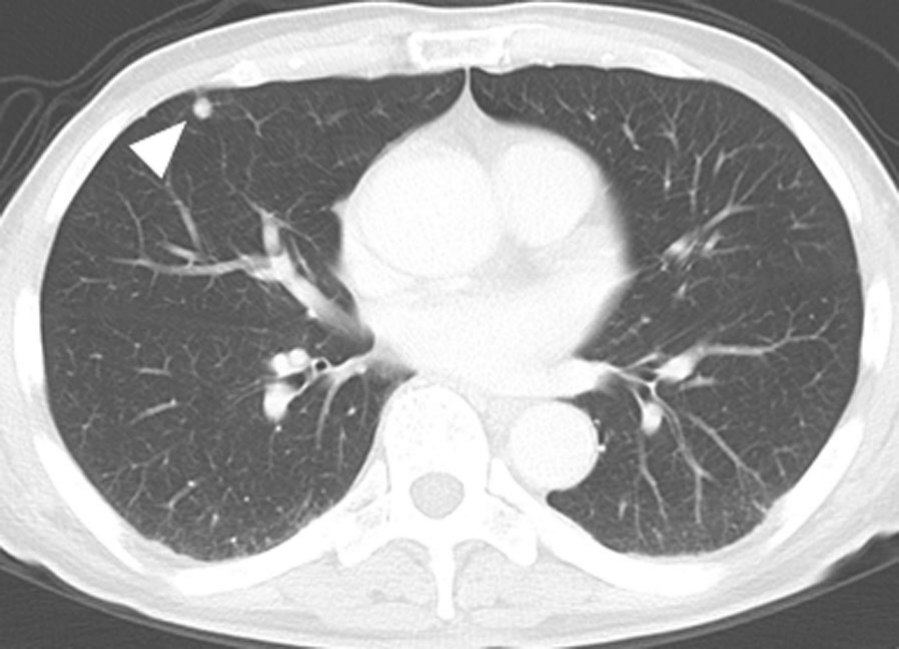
Computed tomography of the lung revealed a 6-mm-size nodule (white arrowhead) in the right middle lobe
